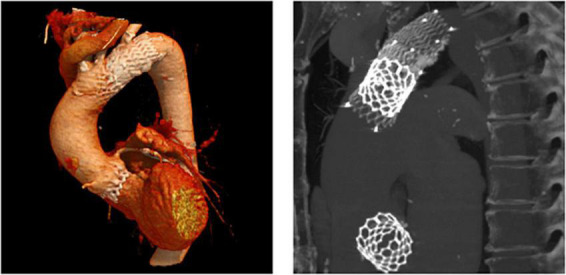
CT images of a patient with embolization of a Sapien 3. CT follow-up of patient number 7 (Table 5). After embolization due to loss of capture during implantation, the embolized Edwards Sapien 3 was pulled back into the proximal aortic arch by the semi-inflated delivery balloon and affixed using two self-expanding stents.
